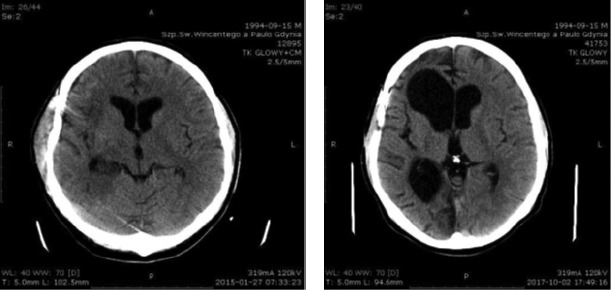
Head CT scans, January 27^th^, 2015 and October 2^nd^, 2017
